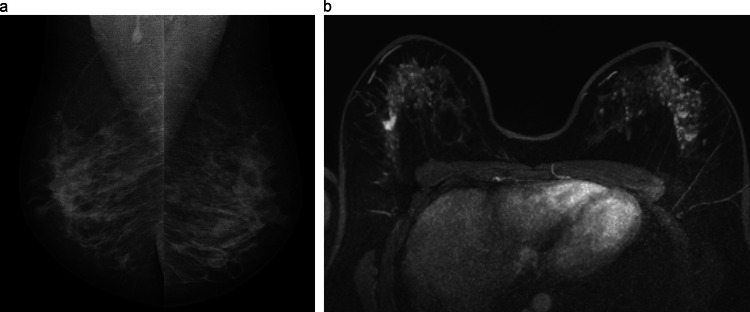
46-year-old patient with a prior history of chest irradiation for Ewing sarcoma. Screening examinations. MLO views (a) from mammography examination that was deeemed normal. Contrast-enhanced breast MRI (b) reveals bilateral breast abnormalities. In the right breast, an irregular spiculated mass is visible, corresponding to a 13 mm NST carcinoma. In the left breast, segmental heterogeneous nonmass enhancement is seen, corresponding to extensive DCIS with microinvasion
